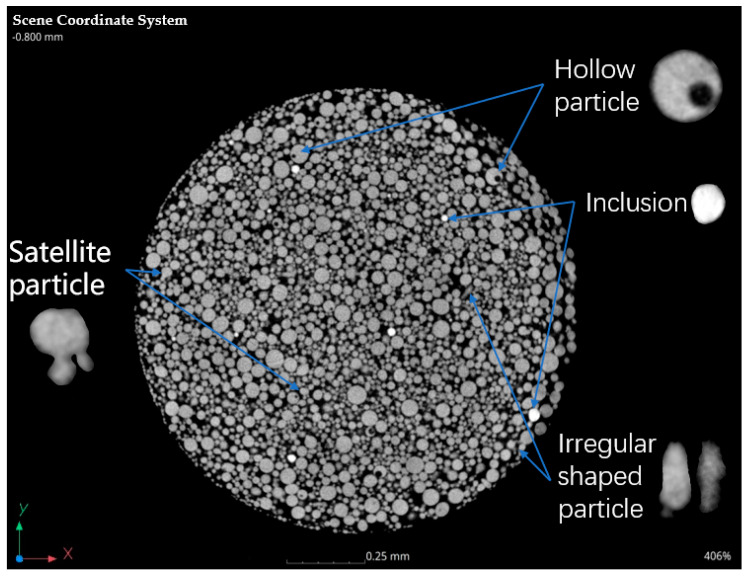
Information shown in the 2D CT image.
